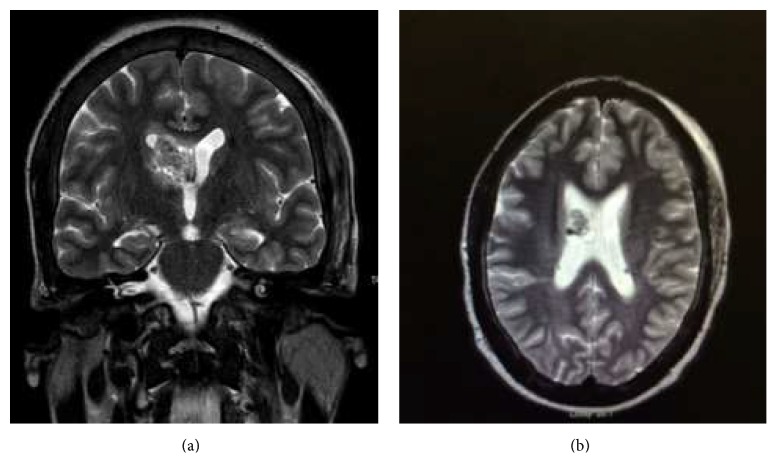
T2 weighted coronal (a) and axial (b) MRI showing 23 × 23 × 18 m lobulated mass in the right lateral ventricle and roof of the third ventricle which is isointense to grey matter and has small signal voids in keeping with calcifications.
